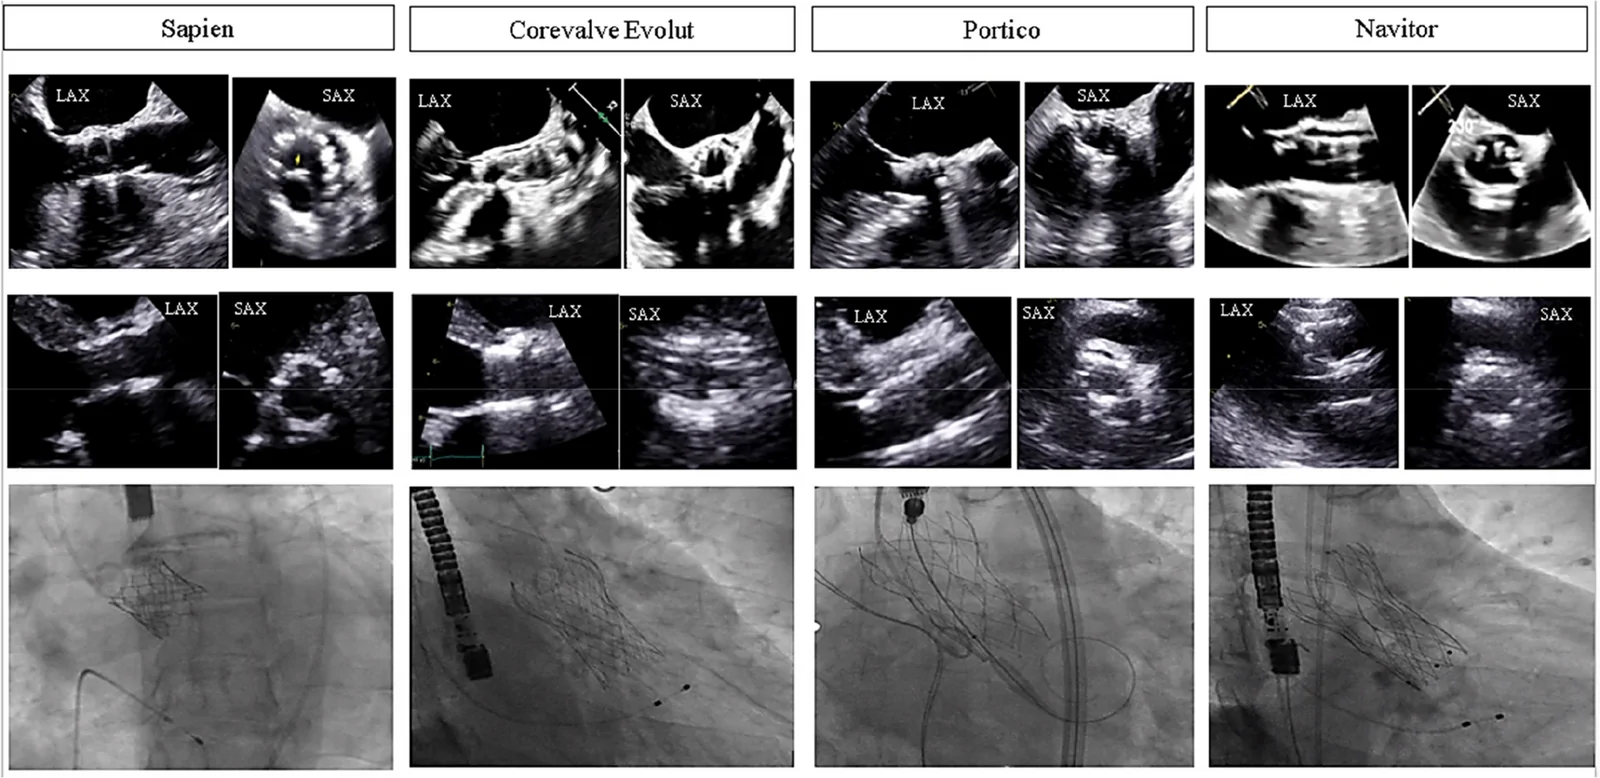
Echocardiographic and fluoroscopic appearance of the various transcatheter aortic valve implantation (TAVI) valves. Examples of commercially available TAVI valves (from balloon-expandable to self-expanding platforms) with normal valve function. First row, transesophageal echocardiography imaging; middle row, transthoracic echocardiography imaging; bottom row, fluoroscopy. LAX, long-axis; SAX, short-axis
